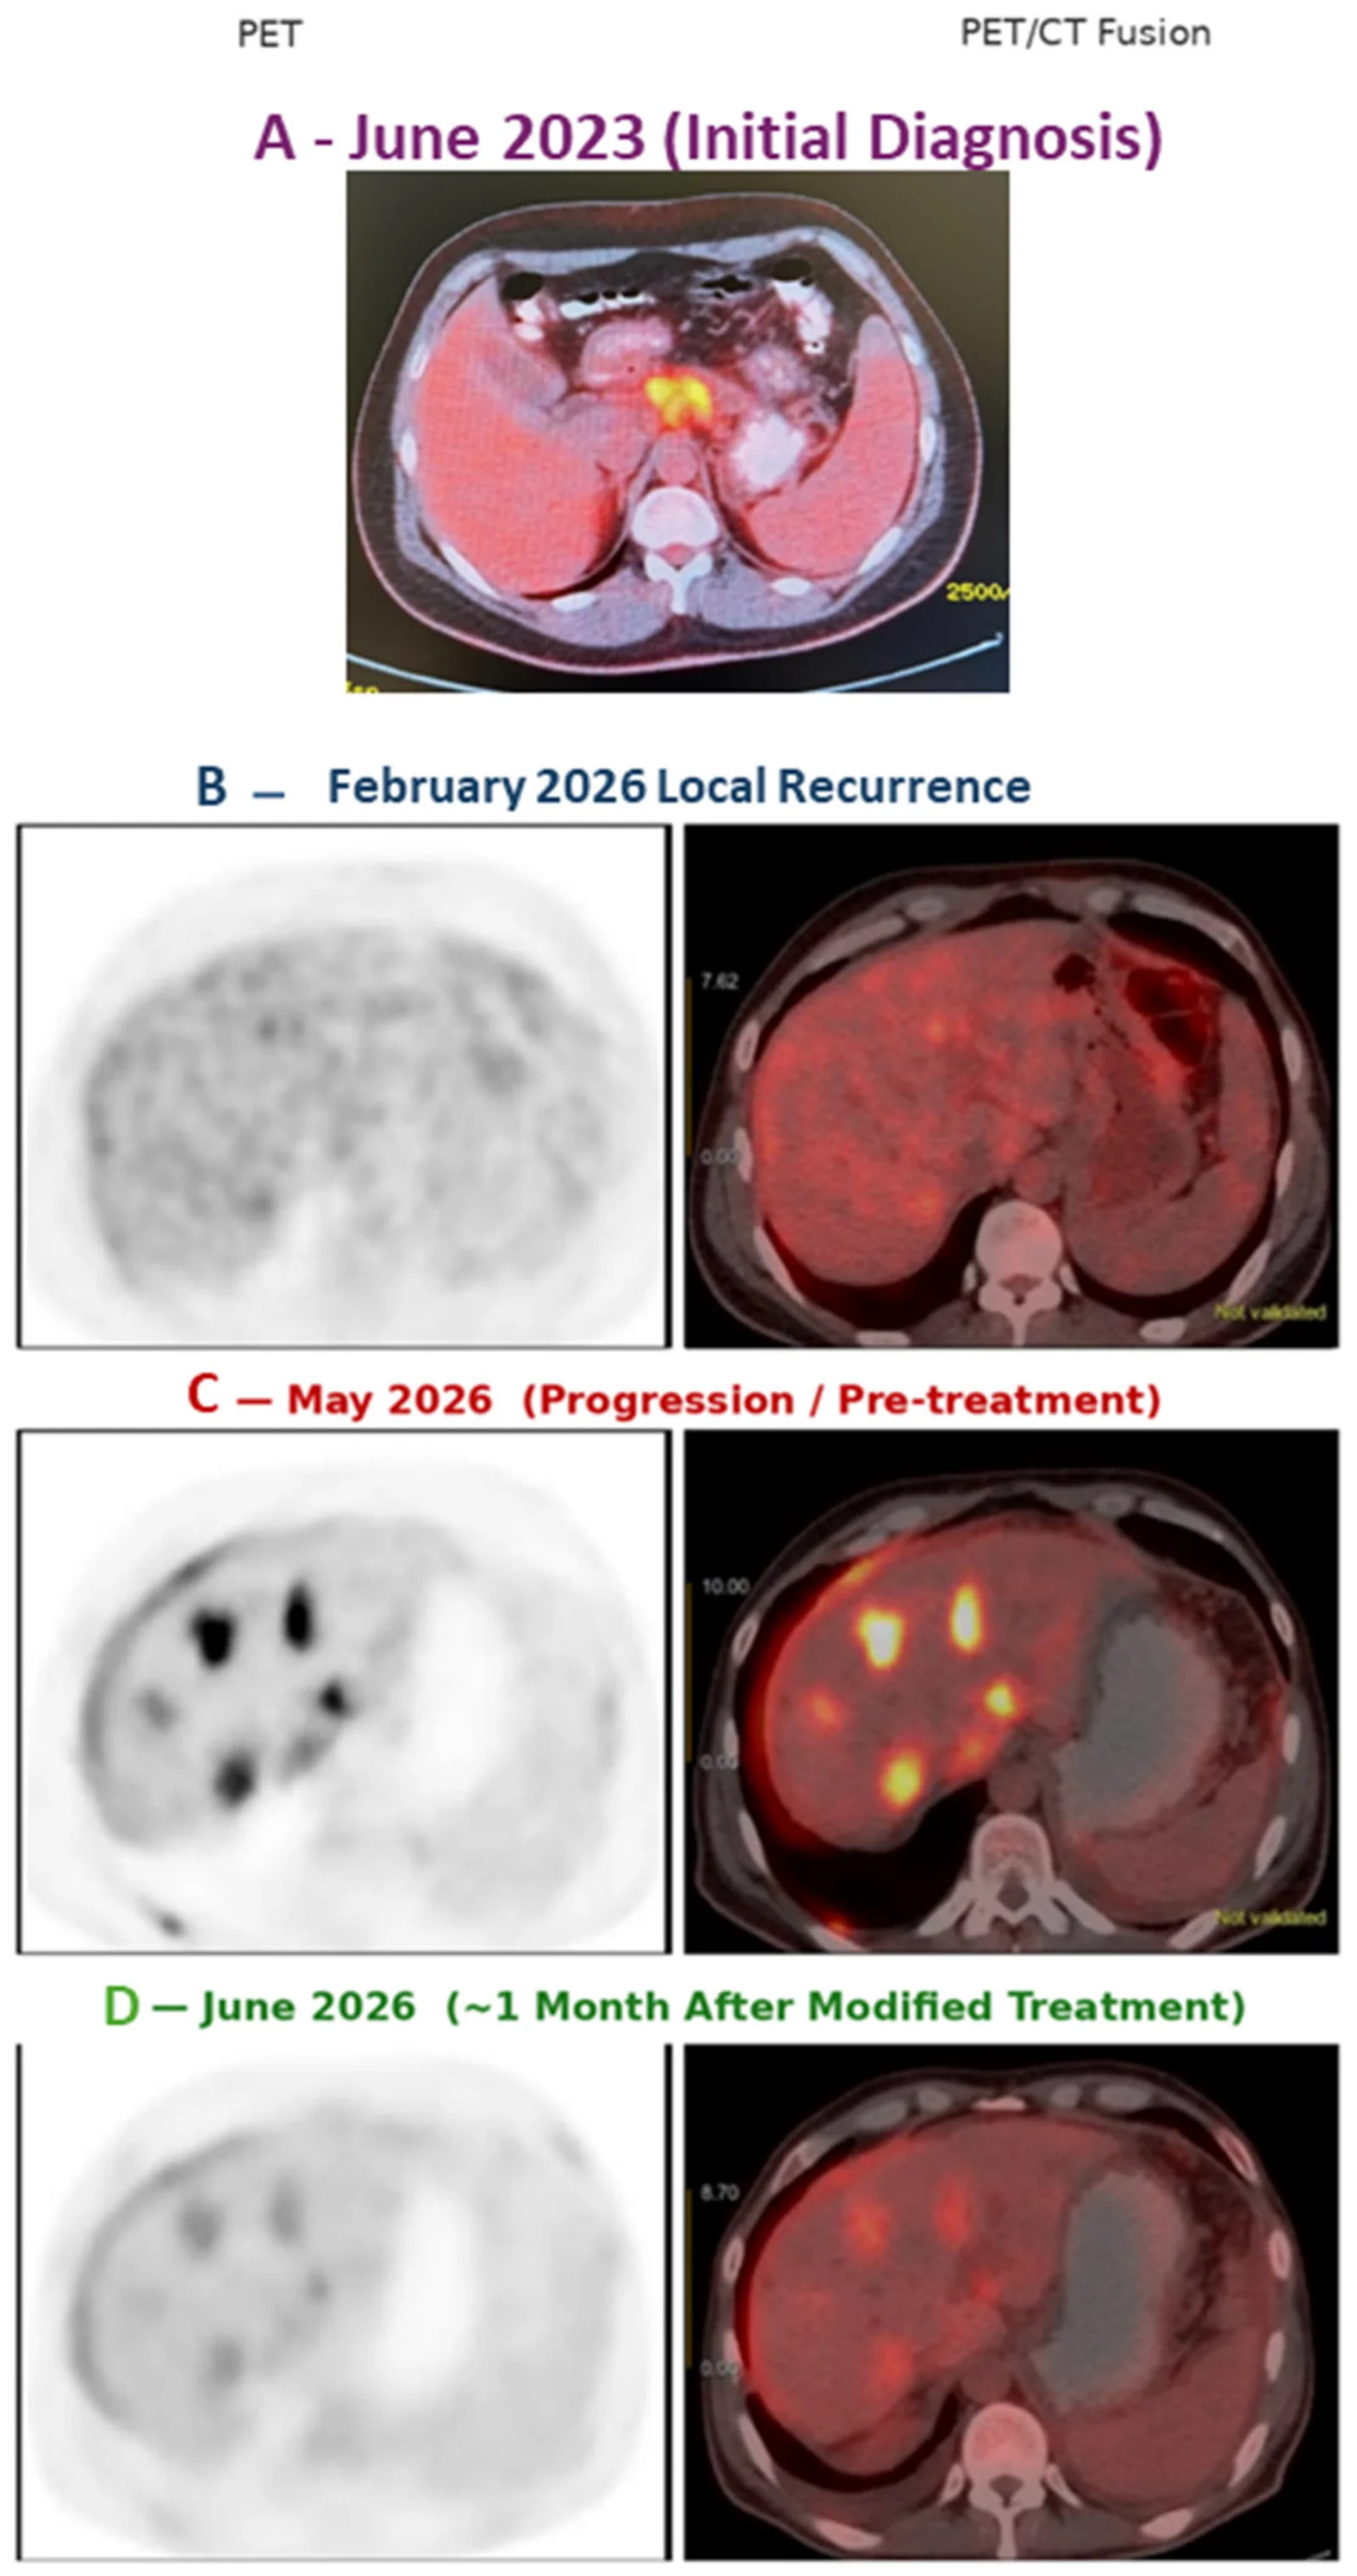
Serial 18F-FDG PET/CT imaging during the patient’s clinical course. (A): baseline PET/CT at diagnosis (June 2023) demonstrating FDG uptake in the primary pancreatic lesion (SUVmax 2.4). (B): PET/CT performed at local recurrence (February 2026). (C): PET/CT at disease progression before treatment intensification (May 2026), showing multiple hypermetabolic liver metastases and local recurrence (SUVmax 18.2). (D): PET/CT obtained one month after multimodal treatment demonstrating a marked reduction in FDG uptake (SUVmax 6.6). Formal PERCIST assessment was not possible because SULpeak and standardized liver reference measurements were unavailable.
